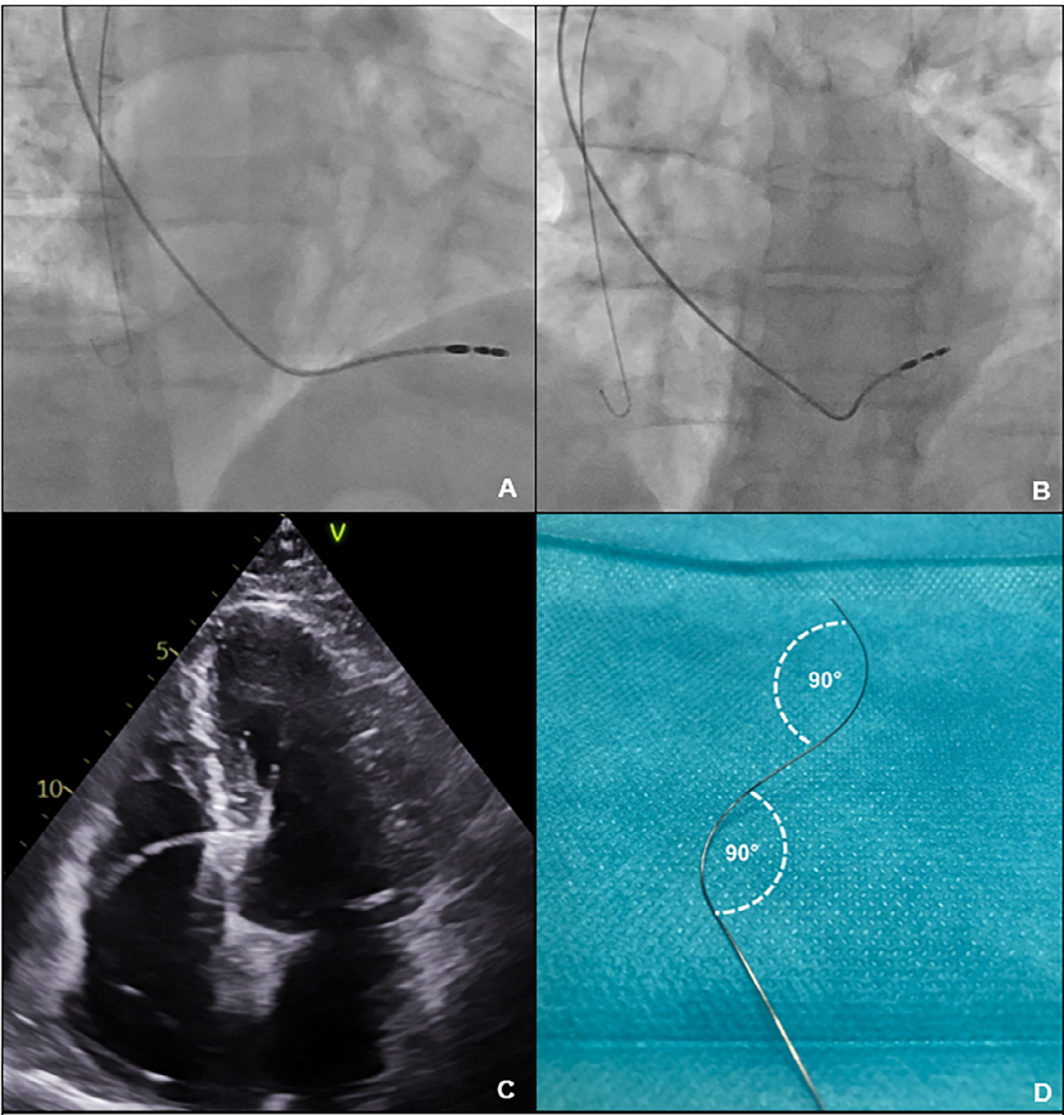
Representative images of LBBAP implantation using an implantation technique with a pre‐bent 3‐dimensional stylet. Fluoroscopy of lead positioning in RAO 20° (A) and LAO 30° (B). (C) Transthoracic echocardiography demonstrating a septal lead position. (D) A manually shaped stylet was used for lead placement. [Colour figure can be viewed at wileyonlinelibrary.com]
